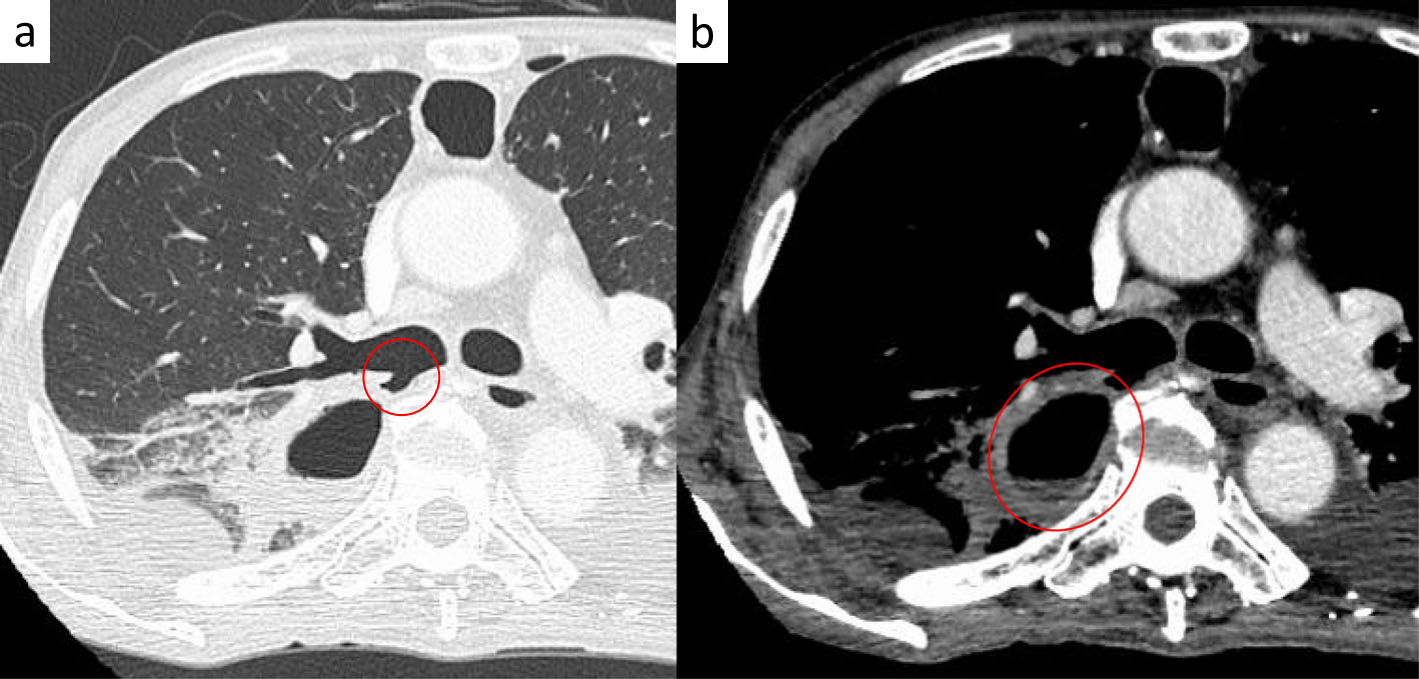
Chest CT scan images showing a a defect in the membranous portion of the right main bronchus and b an air space and air–fluid level near the right main bronchus
